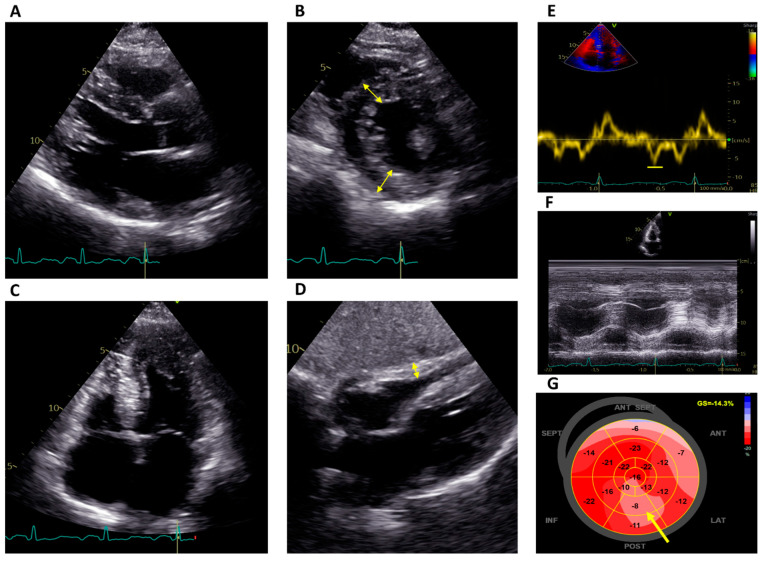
Echocardiographic features of a patient with Fabry disease on ERT. (A–C) Parasternal long-axis view, short-axis view at the level of the papillary muscles and four-chamber apical view, respectively, showing concentric left ventricular hypertrophy (double-edged arrows; septum 17 mm) with hypertrophy of the papillary muscles. (D) Subcostal four-chamber view demonstrating RV hypertrophy (double-edged arrow). (E) Reduced tissue Doppler septal strain e’ wave (6 cm/s). (F) Normal RV function (TAPSE 23 mm). (G) Reduced global longitudinal strain (−14.3%) with a regional reduction in the basal and mid inferolateral segments (arrow). ERT: Enzyme replacement therapy; RV: right ventricular.
